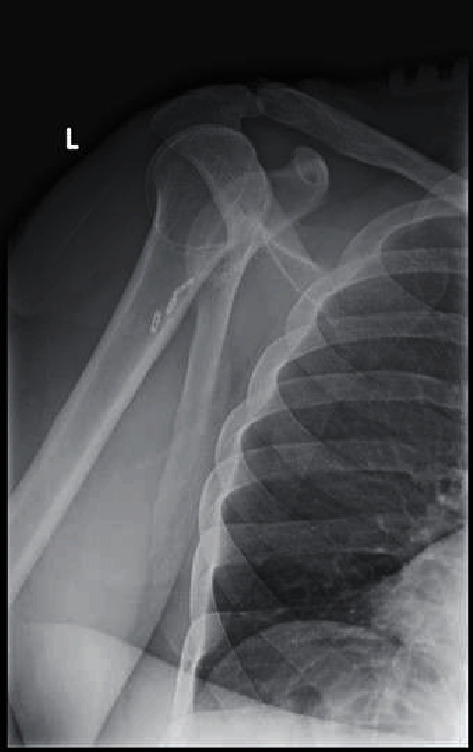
Postoperative radiography demonstrating anchor placements.
